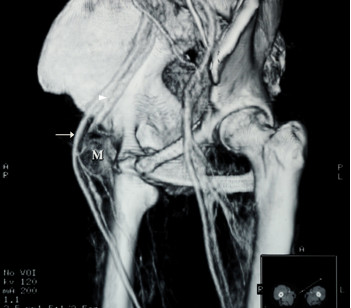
Pre-operative CTA showing a pelvis mass (M) compressing the right femoral artery (arrow) and especially the femoral vein (arrowhead).
